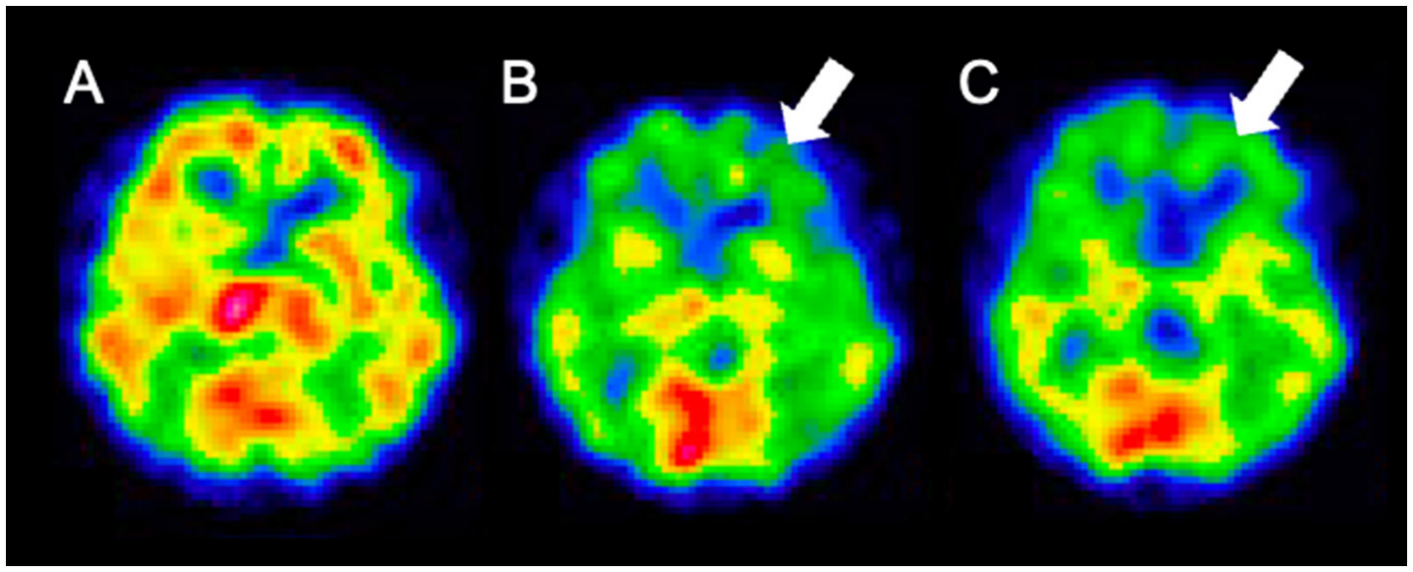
^123^I-IMP SPECT findings. (A) day 12; (B) day 96; (C) day 164. The frontal lobe hypoperfusion is apparent on day 96 and persists. ^123^I-IMP SPECT, N-isopropyl-p-[123I]- iodoamphetamine single-photon emission computed tomography.
